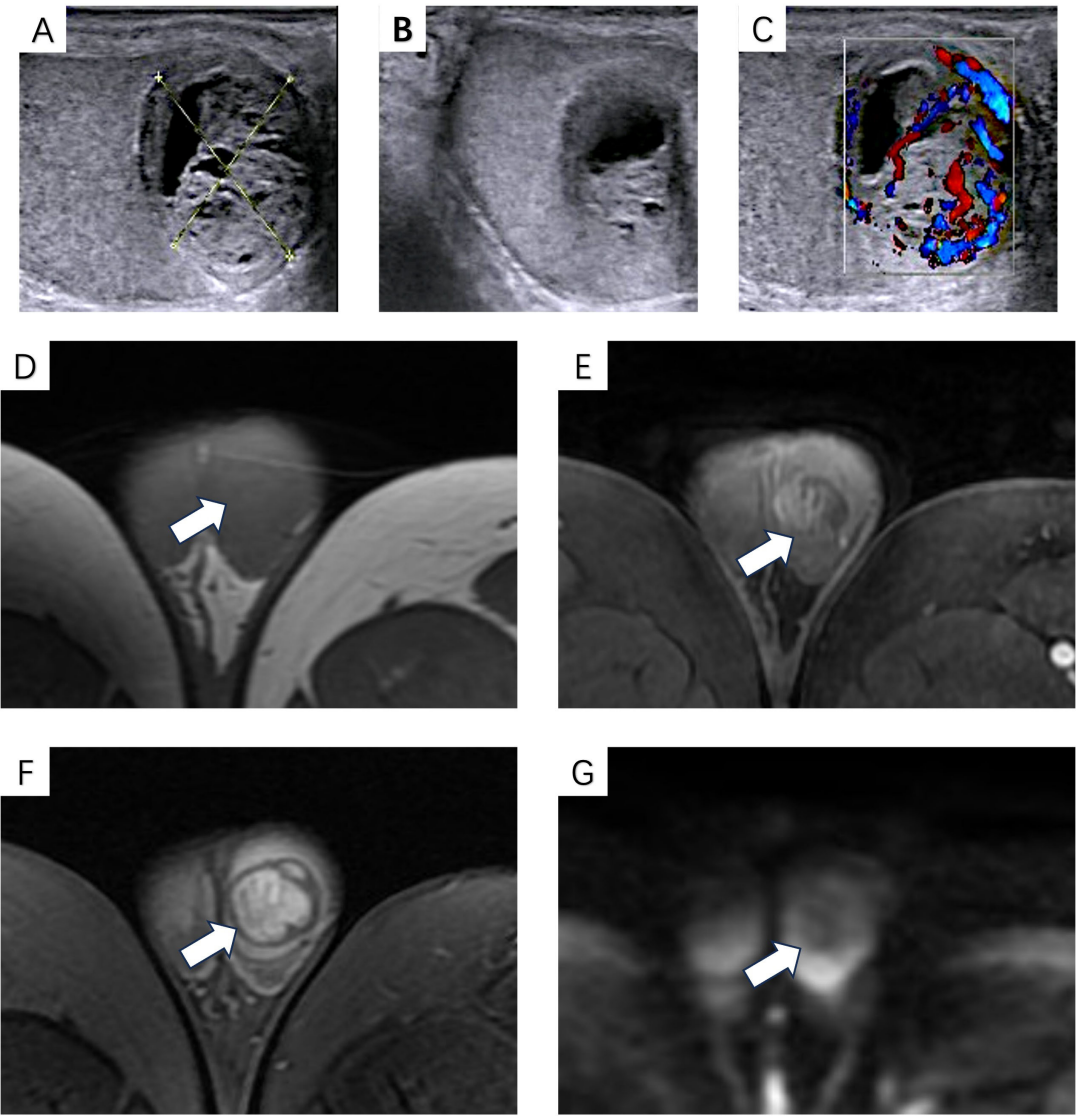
B-mode ultrasound showed a well-circumscribed, regular, solid-cystic mass located within the left testis; the solid component of the mass was predominant, and its rim is slightly hyperechoic (A, B). Color Doppler imaging (CDFI) demonstrated peripheral and intratumoral blood flow signals (C). MRI showed isointense signals on T1-weighted images (D) and heterogeneous signals on T2-weighted images (E), with heterogeneous enhancement in the arterial phase (F); no hyperintense signal was observed on DWI (G).
